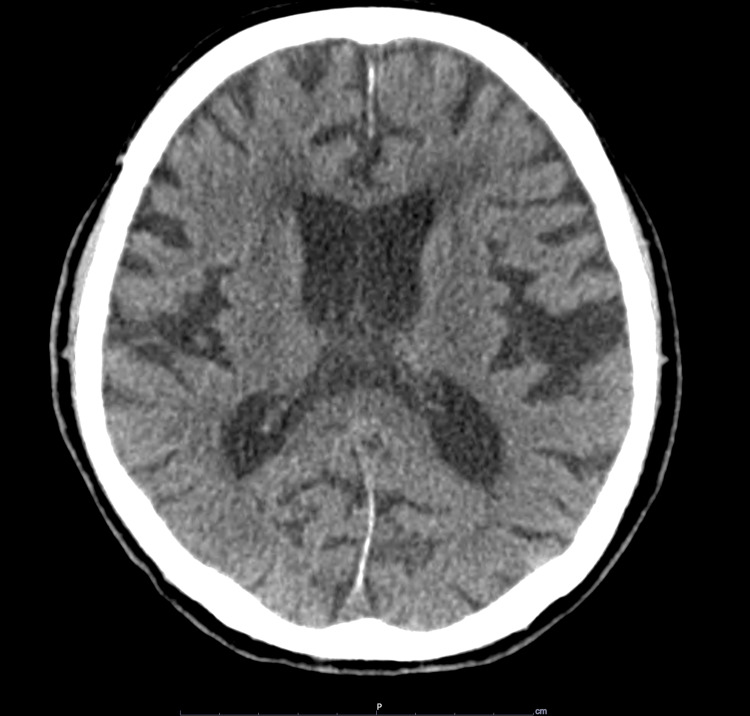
Cross-sectional view of computed tomography of the head without contrast.
